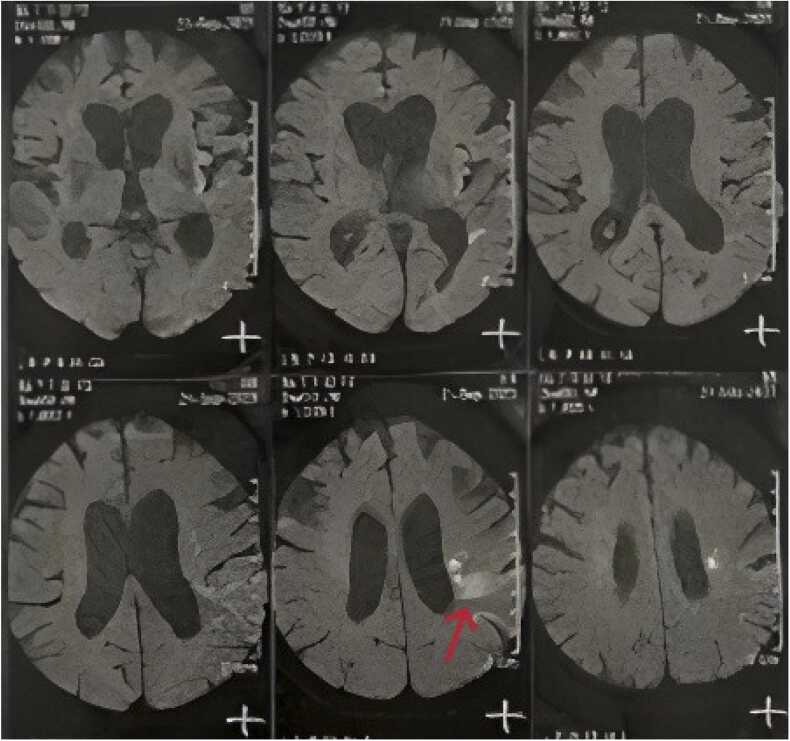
Brain MRI: periventricular, left side centrum semi oval white matter and post central gyrus lesions mostly in favor of microvascular occlusive ischemia in the MCA territory (e.g., lenticulostriate artery) - mild cortical atrophy and ventriculomegaly are also noted.
